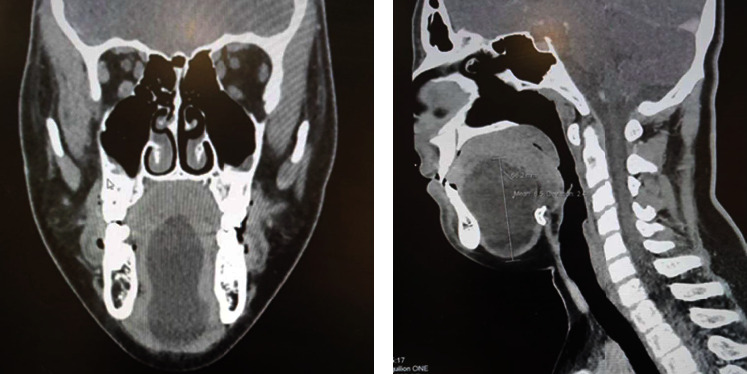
(a) Coronal and (b) sagittal CT scans revealing a homogeneous cystic structure measuring 6.5 cm in diameter with a delicate capsule without evidence of tissue component in the floor of the mouth, located centrally and anteriorly to the hyoid bone and below the tongue.
